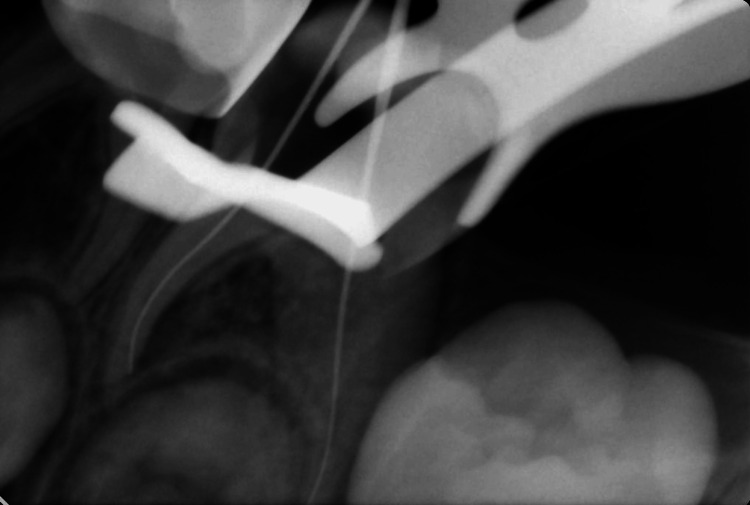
Periapical radiograph to confirm working length
